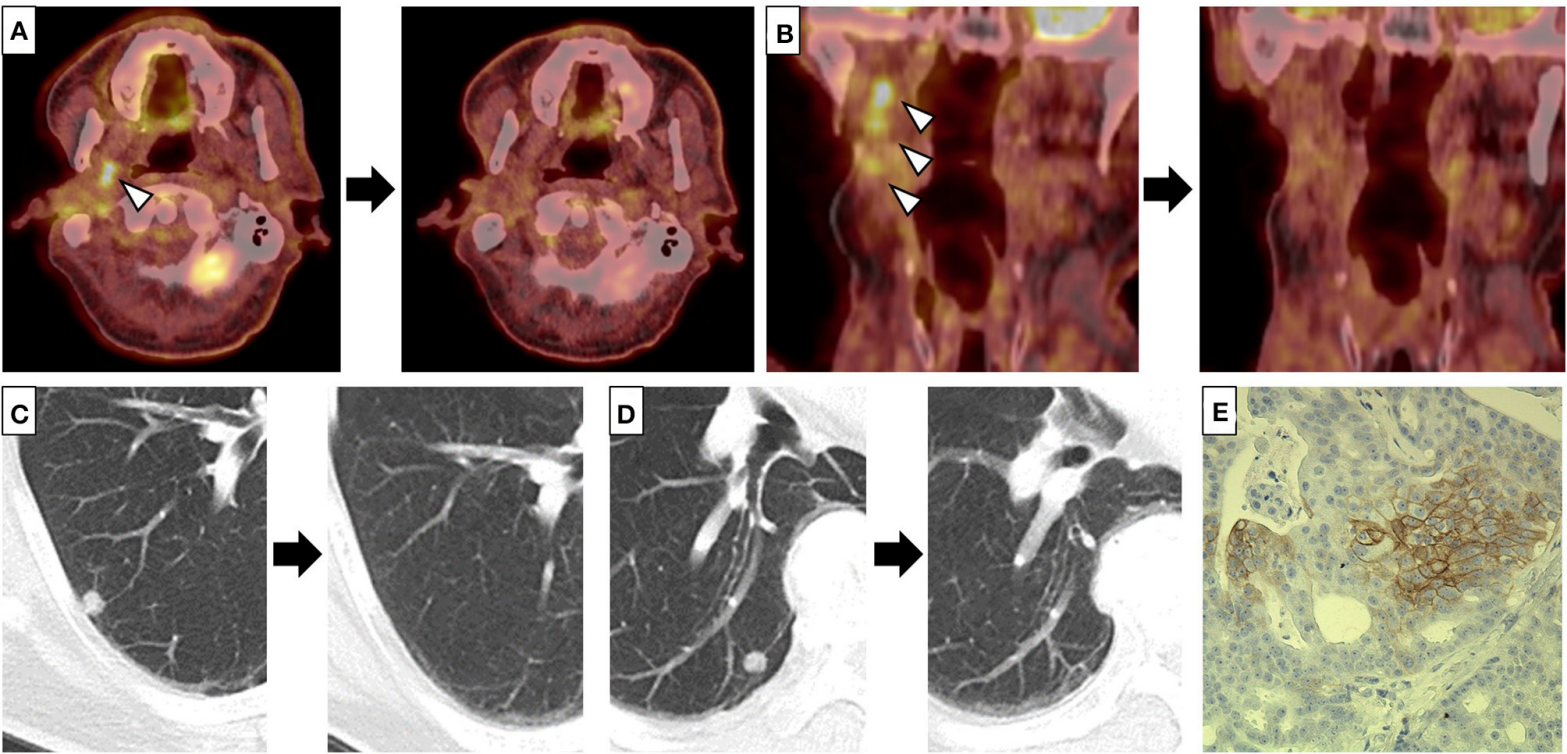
(A–D) Fluorodeoxyglucose (FDG) accumulation of the mass below the surgical wound of the parotid gland (A) and right upper deep cervical lymph nodes (LNs) (B), and multiple lung nodules (C,D) disappeared after a single course of combined nivolumab and ipilimumab therapy. Left and right panels indicate positron emission tomography-computed tomography (PET-CT) (A,B) or CT (C,D) before and 4 months after a single course of combined therapy, respectively. Arrow heads indicate the mass below the surgical wound of the parotid gland (A) and the cervical LNs (B) with high FDG accumulation, respectively. (E) Some of the tumor cells of the recurrent cystadenocarcinoma were positive for PD-L1 (clone: sp142, ×200).
